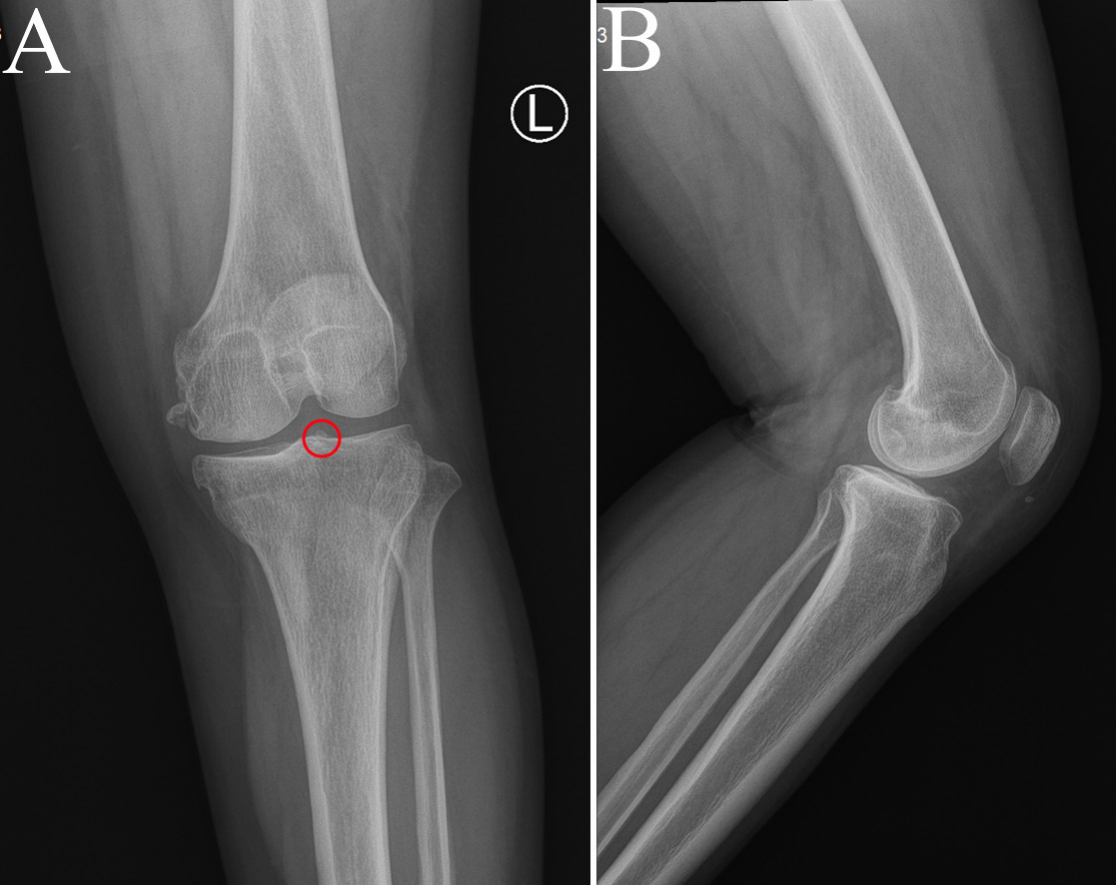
X-ray scan showing meniscal ossicle (circle).
